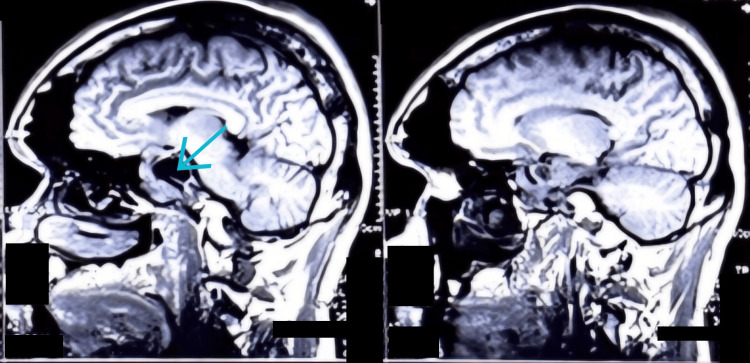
MRI showing pituitary adenoma. Arrow pointing at the necrotic/cystic center.
